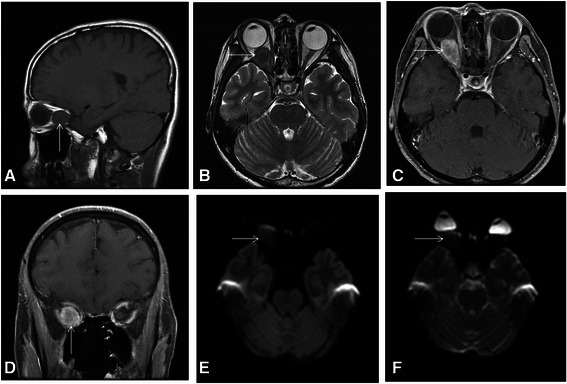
Intraorbital granular cell tumor on orbital magnetic resonance imaging. The tumor shows isointensity on a the sagittal T1-weighted image relative to gray matter and hypointensity on b the axial T2-weighted image. Diffuse heterogeneous enhancement with intravenous administration of gadolinium is noted on c axial and d coronal images. In c and d, the tumor appears in close association with inferior, lateral, and medial rectus muscles and the right optic nerve. The arrows are pointing to the tumor within panels a to f. On e diffusion-weighted image (*b* value = 1000) and f apparent diffusion coefficient map, the tumor shows isointensity relative to normal brain tissue without diffusion restriction
